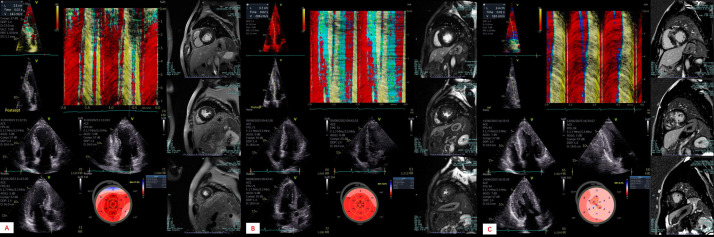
Ultrasound imaging in HCM fibrosis assessment. (A) Non-fibrotic HCM: LGE negative, SWV = 143 cm/s, GLS = –17.8%, normal tissue elasticity, mildly reduced systolic function. (B) Mild fibrosis HCM: LGE positive, focal distribution, SWV = 206 cm/s, GLS = –12.9%, elasticity elevated, strain decreased. (C) Severe fibrosis HCM: LGE positive, extensive distribution, SWV = 310 cm/s, GLS = –5.2%, spasticity significantly elevated, strain severely impaired. Source: images from our hospital’s cardiovascular surgery department from 2022 to 2024. HCM, hypertrophic cardiomyopathy; GLS, global longitudinal strain; LGE, late gadolinium enhancement; SWV, shear wave velocity.
